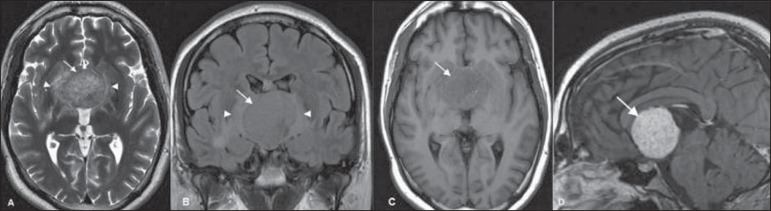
Axial MRI T2-weighted (A) and coronal FLAIR (B) sequences
